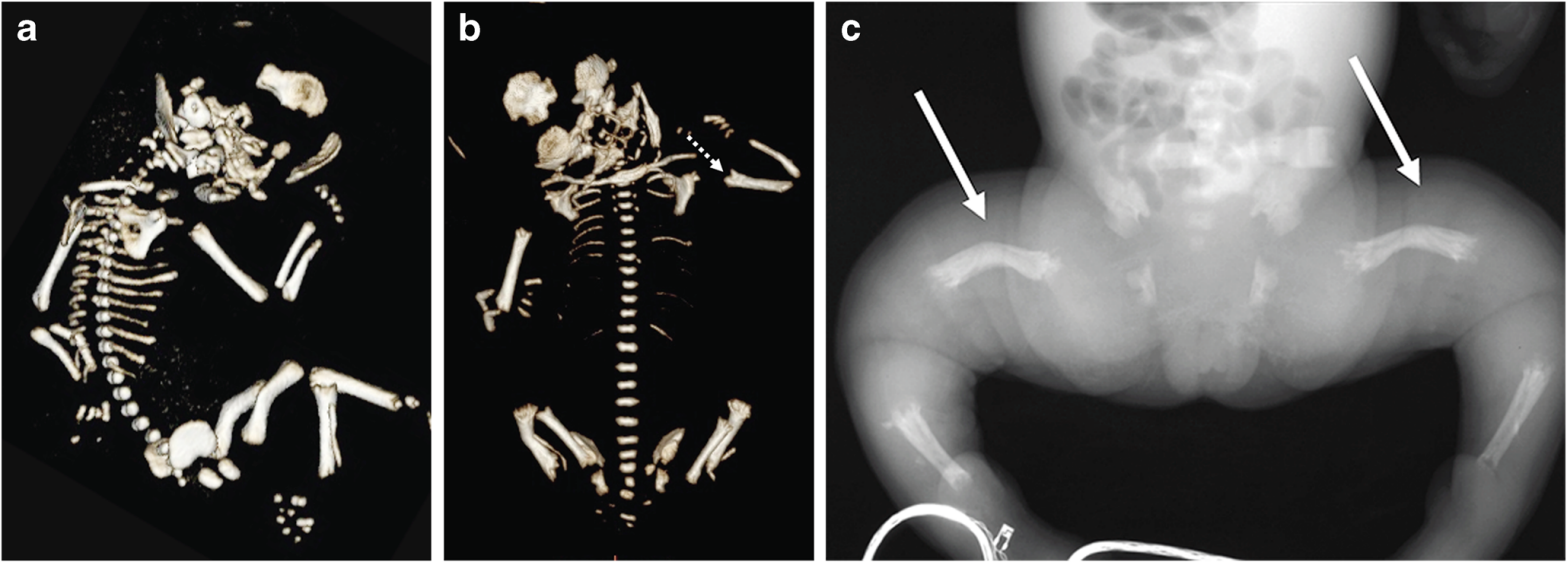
Imaging features of hypophosphatasia in a fetus at 25, 34, and 38 weeks’ gestation*.* The fetus died. a,b Three-dimensional reconstructed fetal CT images show deficient ossification of ribs, which is progressive between 25 weeks’ (a) and 34 weeks’ (b) gestation, and wide cranial sutures and anterior fontanelle. Metaphyseal “tongues” of radiolucency are best appreciated on the CT scan at 34 weeks (*dashed arrow*). c Postmortem anteroposterior radiograph of the same fetus at 38 weeks’ gestation shows bowed femora (*arrows*) and absent ossification of pedicles
